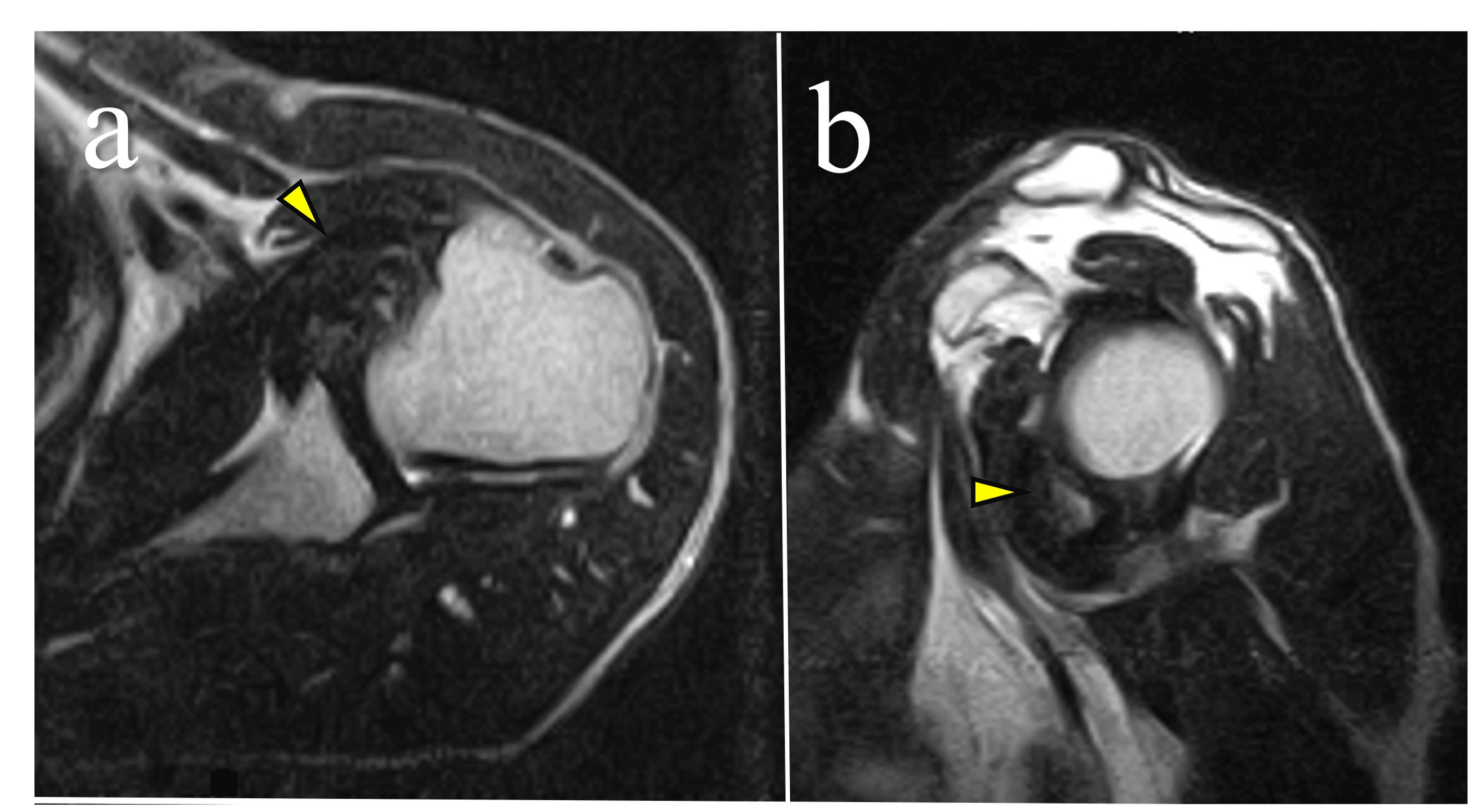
Magnetic resonance imaging obtained six years before referral to our institution. (a) Axial T1-weighted and (b) sagittal T2-weighted images demonstrating a space-occupying lesion deep to the subscapularis muscle (arrowheads) with low signal intensity on both sequences.
